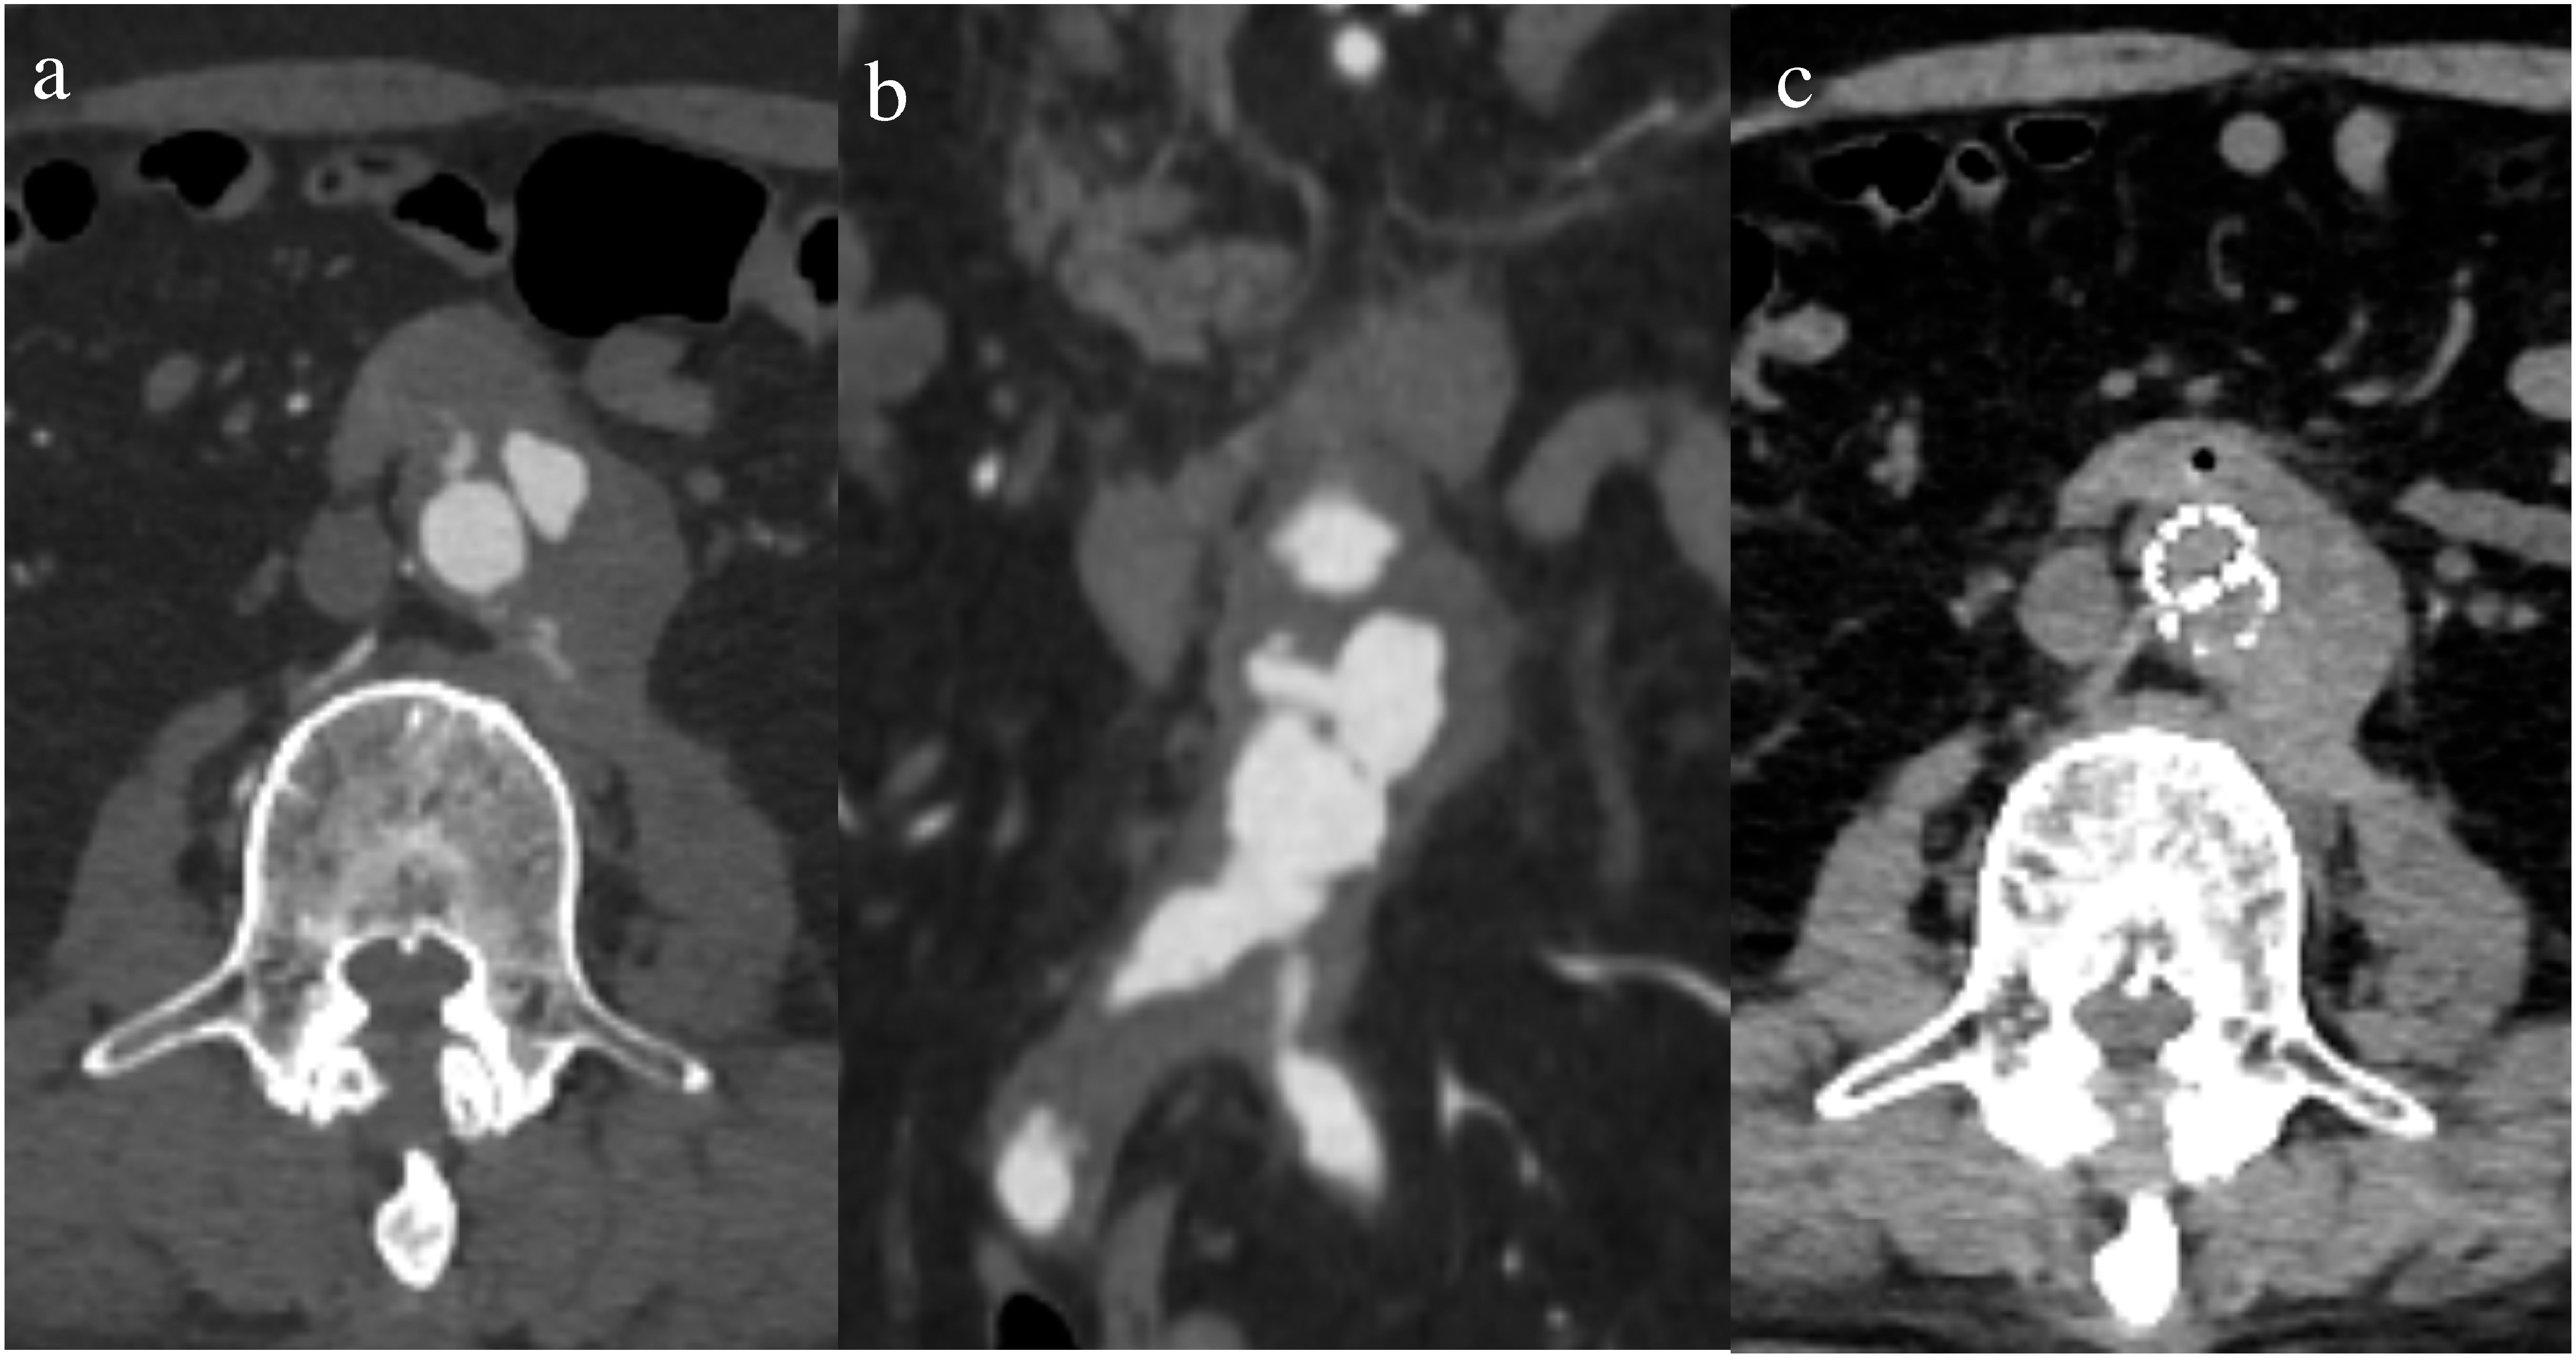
Fig. 1 Computed tomography angiography revealed that the horizontal (a) and coronal (b) portions of the duodenum were close to an abdominal aortic aneurysm without leakage of Gastrografin after endovascular aneurysm repair (c).
